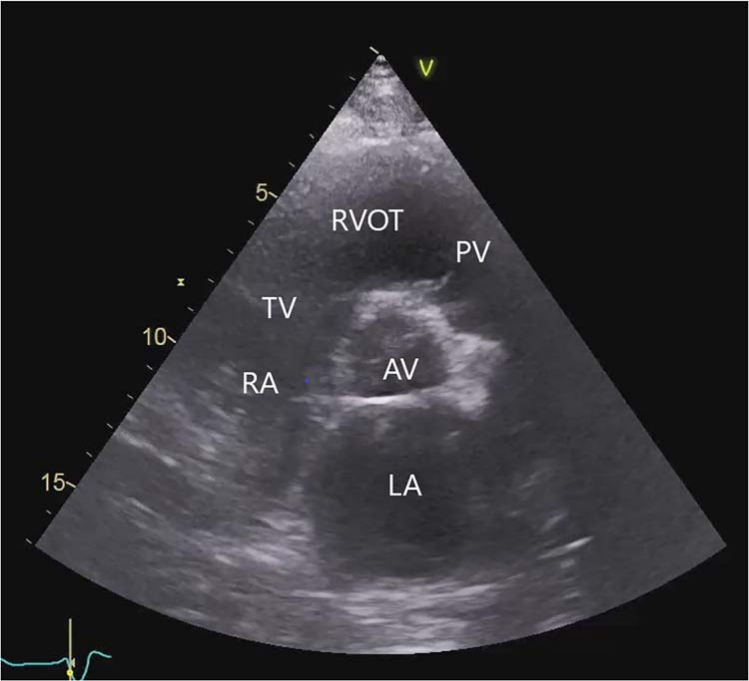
Transthoracic echocardiogram before LVAD implantation. Parasternal short-axis view. AV (aortic valve), LA (left atrium), PV (pulmonary valve), RA (right atrium), RVOT (right ventricular outflow tract), TV (tricuspid valve).
